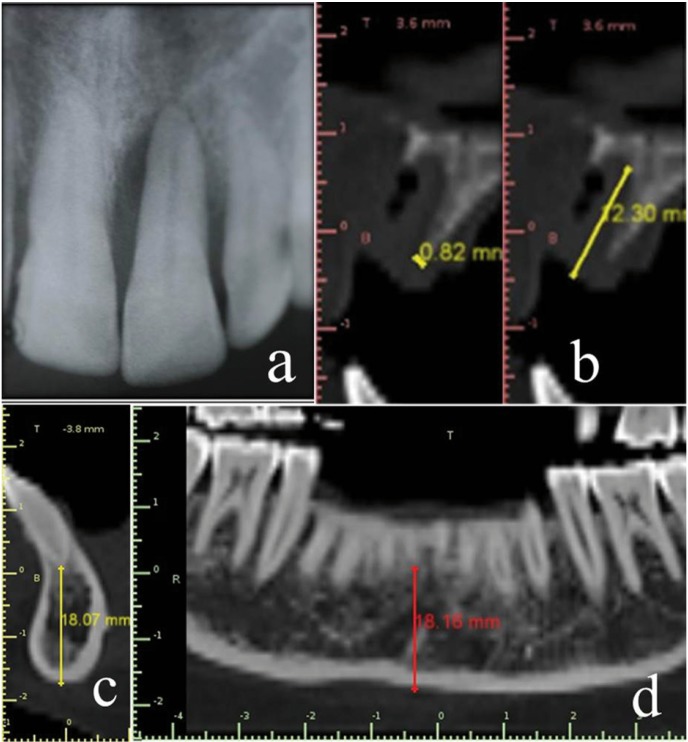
Pre-operative radiographic view of the recipient and donor site a) Periodontally hopeless tooth. b) Height and width of the deficient ridge immediately after extraction. c,d) Determination of safety outline for chin graft harvest.
